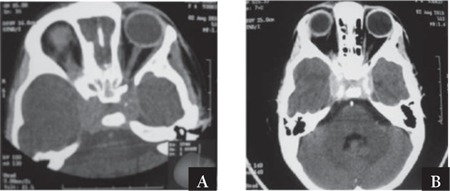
Contrast-enhanced and non-contrast-enhanced axial cranial CT scans. A. Mild hyperdense lesion in the sellar region with well-defined borders. B. Homogeneous significant contrast enhancement and extension of the lesion to the cavernous sinus is seen following intravenous injection of contrast material.
